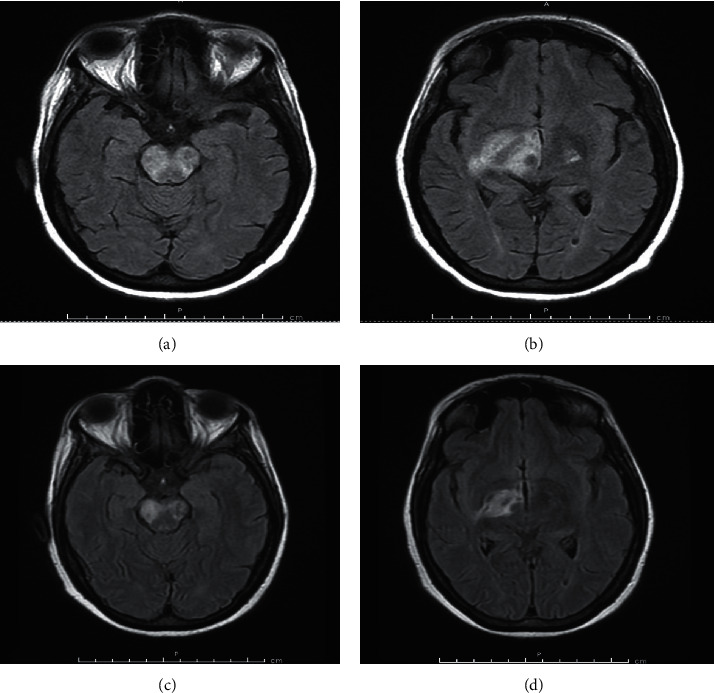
FLAIR brain MRI of a 52-year-old African American woman with active SLE demonstrating edematous lesion in the brainstem (a) extending to the basal ganglia (b) at initial hospital presentation in July 2019. Edema and lesion size decreased posttreatment with methylprednisolone, PLEX, and cyclophosphamide (c) and (d) FLAIR fluid-attenuated inversion recovery.
